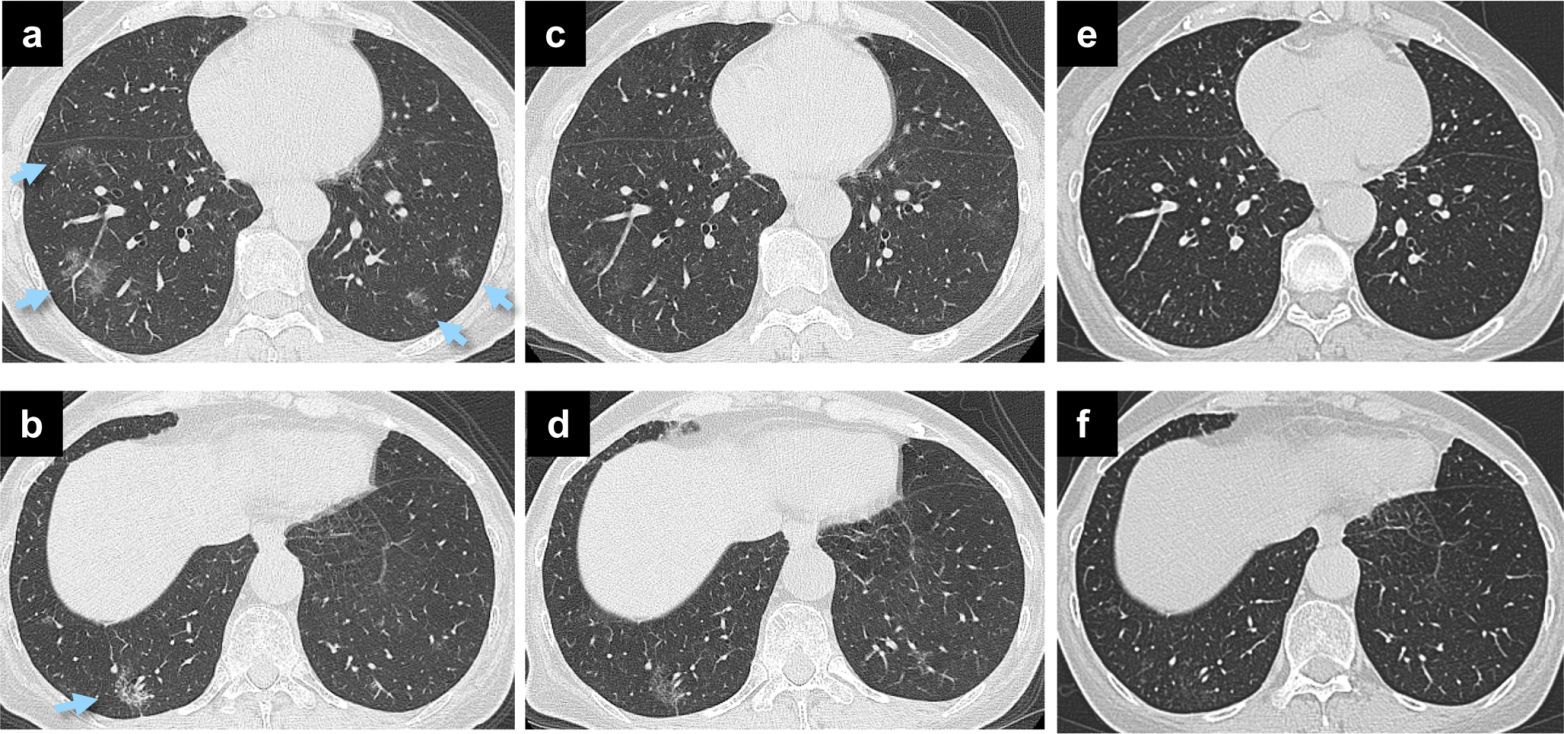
Chest computed tomography scans. Two months before the initial visit (a) multiple ground‐glass nodules (arrows) at the bilateral lower lobes and (b) the right S^10^ nodule with a pleural tag (arrow) were seen. Ten days after the cessation of vildagliptin and the *Lactobacillus paraplantarum* probiotic supplementation, (c) the multiple ground‐glass nodules had nearly disappeared and (d) the right S^10^ nodule had decreased in density. Four months later, (e) multiple ground‐glass nodules and (f) the right S^10^ nodule had entirely disappeared.
